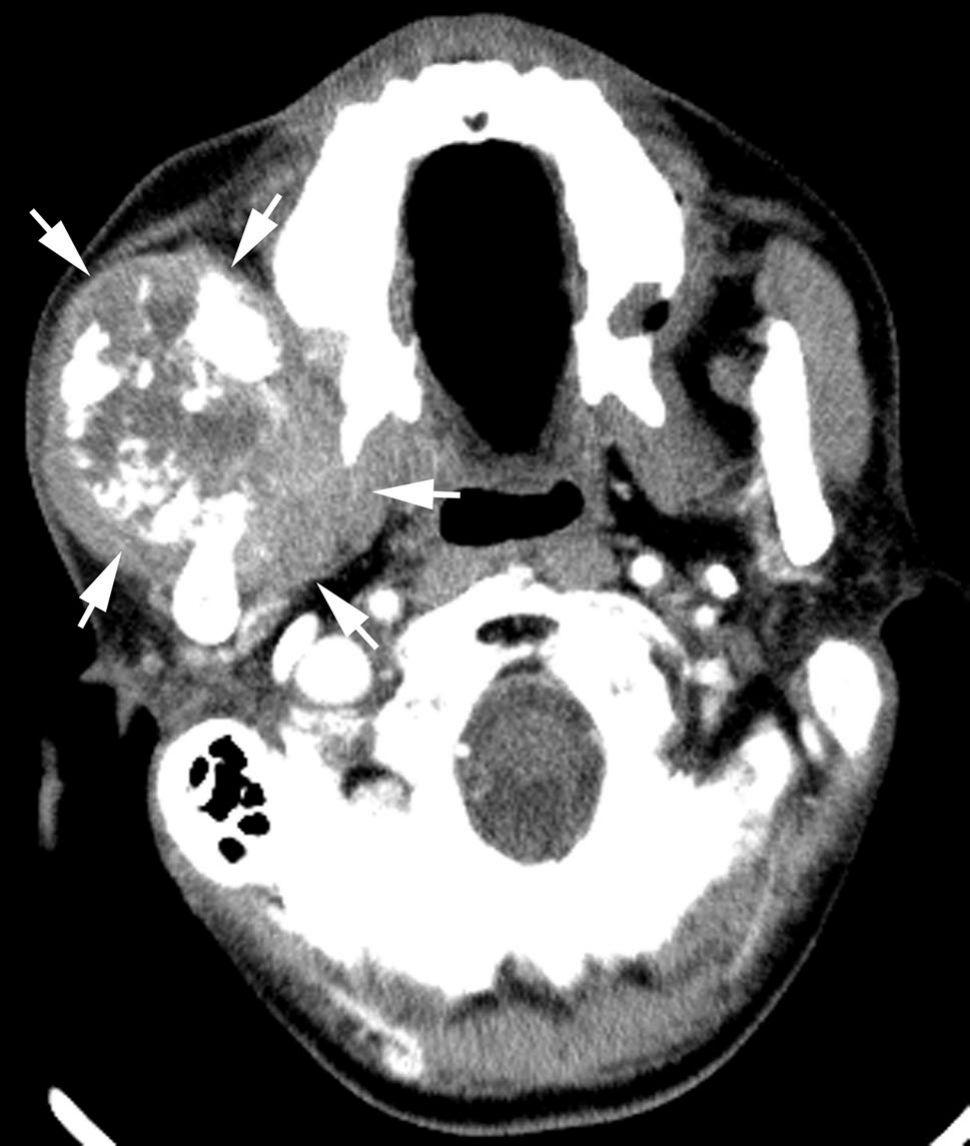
Radiation-induced osteosarcoma 10 years after radiotherapy of a 53-year-old man. Axial contrast-enhanced CT image showed a large heterogeneous tumor, with a large amount of tumor bone formation, arising from the right mandibular ramus destroying the right masseter muscle and medial pterygoid muscle (*arrows*)
